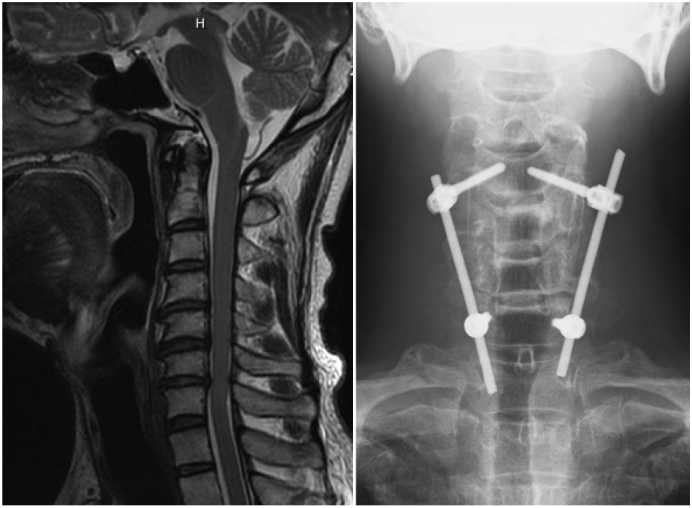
Preoperative magnetic resonance imaging and postoperative radiograph of cervical spine.
